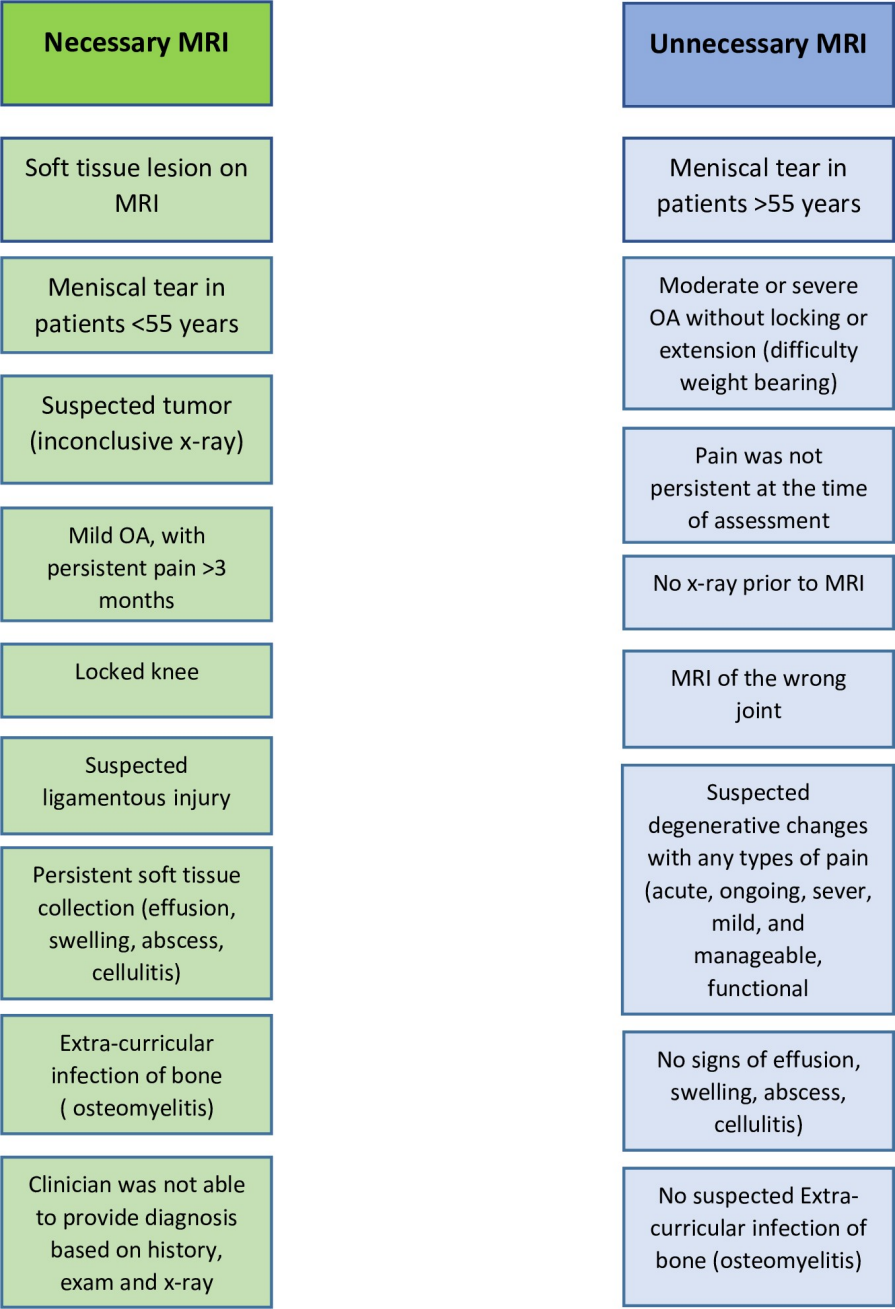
Algorithm to determine necessity of ordered knee pain MRI scans.
